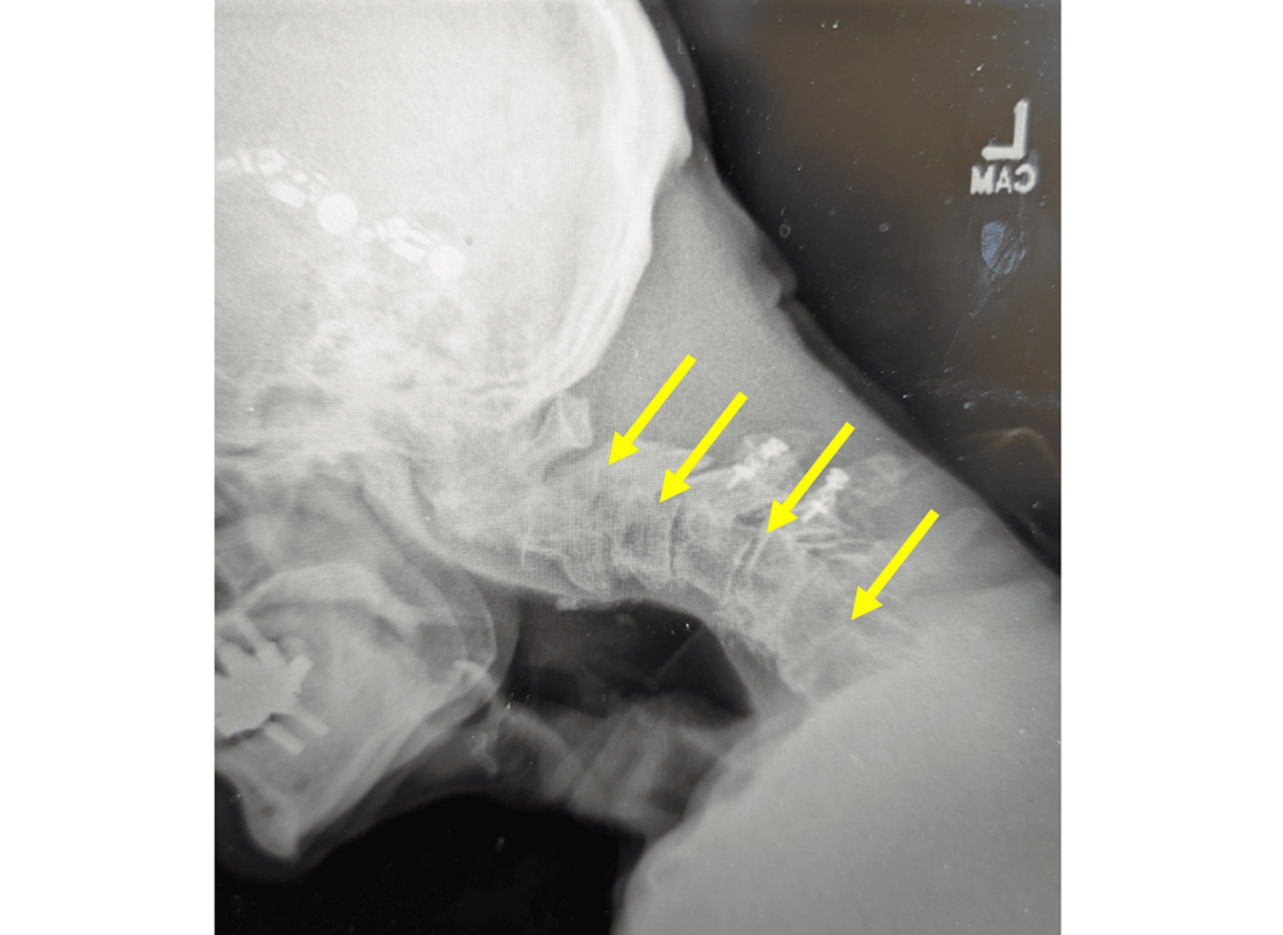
Cervical X-ray findings revealed multilevel degenerative changes (indicated by yellow arrows) without evidence of fracture.
